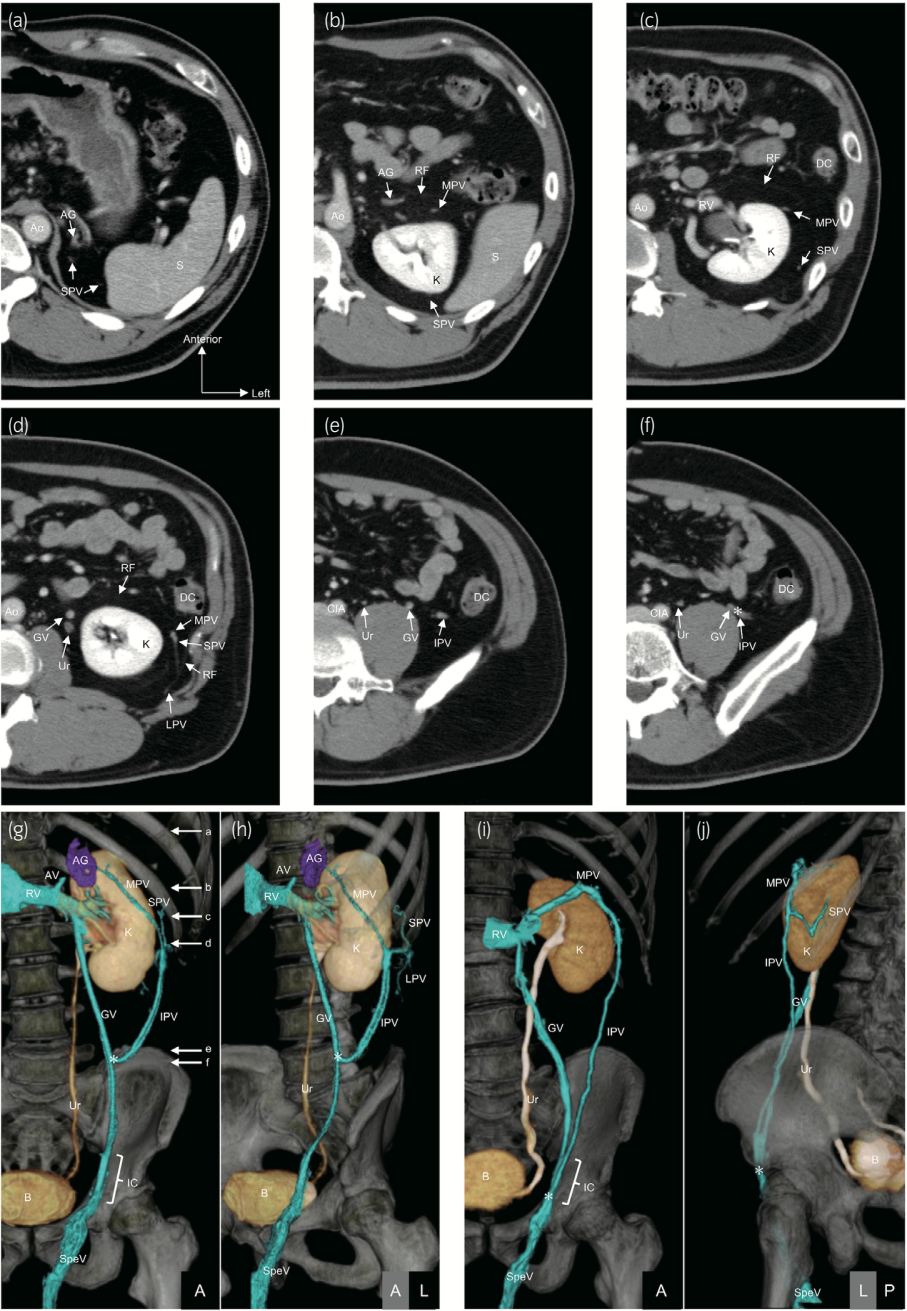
CT images of perinephric veins. (a–f) CT images (axial view) of a 45‐year‐old man. The IPV connected to the GV (asterisk). (g,h) 3‐D reconstructed CT images of a 45‐year‐old man (the same patient in a–f; arrows a–f in g correspond to the section in a–f). The MPV connected to the left emissary vein of the AG and the IPV to the GV at the lower pole of perirenal fat (asterisk). The superior and LPVs were poorly reconstructed. (i,j) 3‐D reconstructed CT images of a 60‐year‐old man. The MPV connected to the left RV and the IPV to the GV in the IC (asterisk). The superior and LPVs were poorly reconstructed. AG, adrenal gland; Ao, aorta; AV, adrenal vein; B, bladder; CIA, common iliac artery; DC, descending colon; GV, gonadal vein; IC, inguinal canal; K, kidney; RF, renal fascia; RV, renal vein; S, spleen; SpeV, spermatic vein; Ur, ureter.
